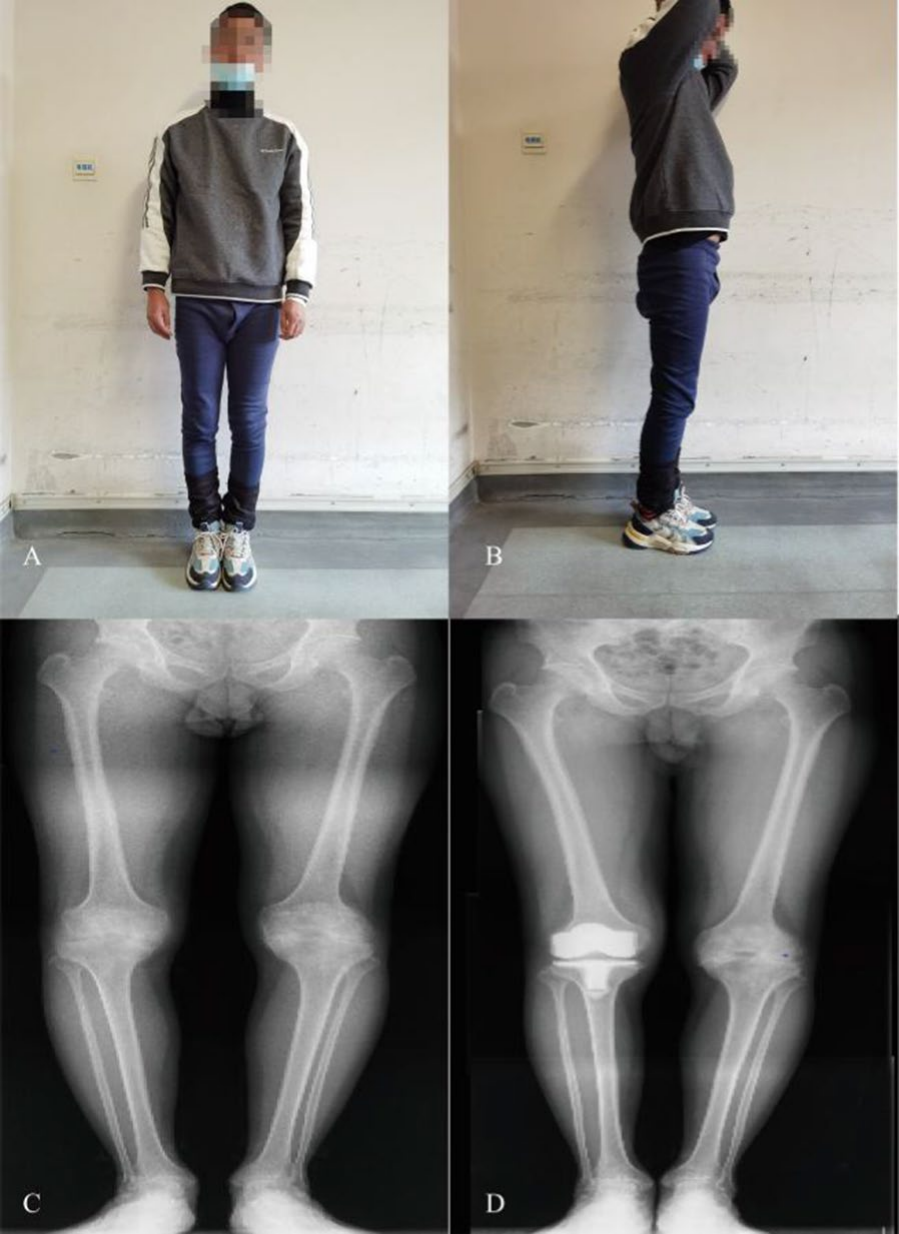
Clinical and radiographic views of both lower extremities. Postoperative X-rays showed a good position of the prosthesis and a good restoration of the force line of the right lower limb
